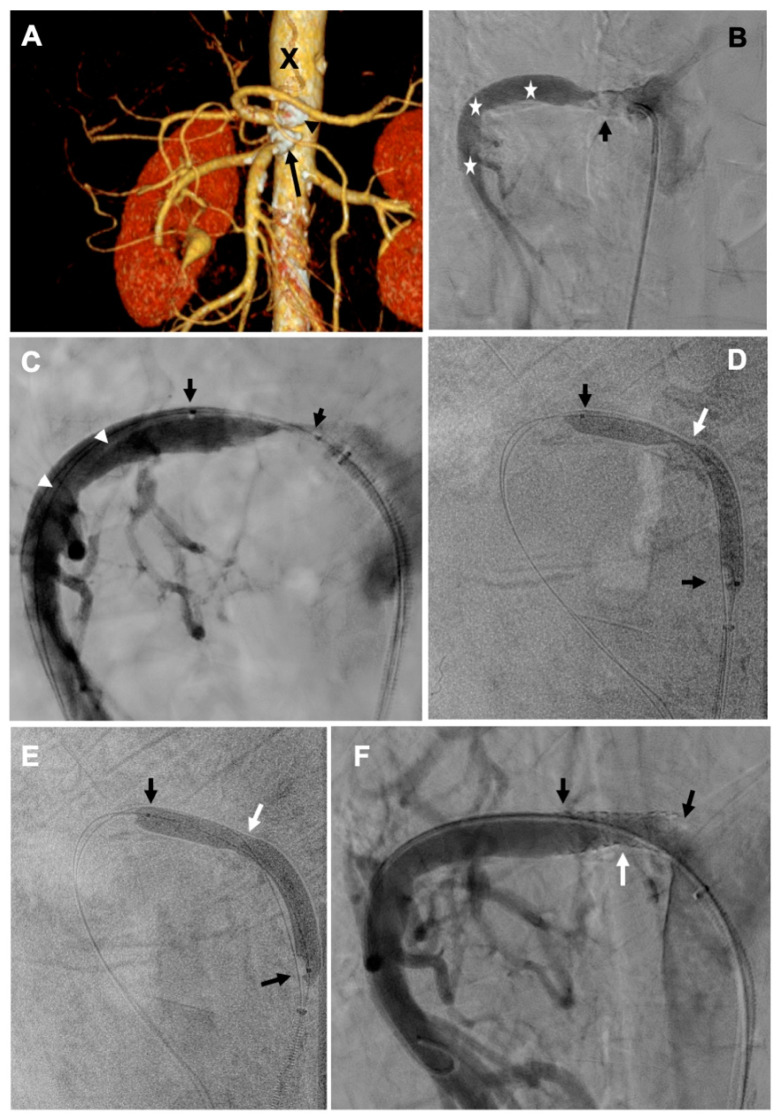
Consecutive steps of revascularization of a narrowed superior mesenteric artery with the use of lithotripsy-assisted angioplasty. (A) CT angiography of the aorta (X) and visceral arteries: massive calcifications in the area of the celiac trunk orifice (arrowhead) and the superior mesenteric artery (arrow); (B) angiography of the superior mesenteric artery (asterisks)—the superior mesenteric artery with critical stenosis in the proximal part and massive calcifications within this lesion (arrow); (C) angiographic result after predilatation with the 3.5/20 and 4.5/20 balloons (balloon between 2 black arrows); balloon angioplasty failed to improve the lesion, and there was >80% residual stenosis; (D) balloon lithotripsy system 6.0/60 Shockwave M5+ (between two black arrows)—before dilation using the lithotripsy system; (E) balloon lithotripsy system 6.0/60 Shockwave M5+ (between two black arrows)—after application of sonic energy and balloon angioplasty: almost complete dilatation of the lesion; (F) final angiographic result: stent visible between two black arrows with a small <5% residual lesion (white arrow; locations of this lesion in subfigures (D,E) are also marked with white arrows) and good inflow to the distal branches.
